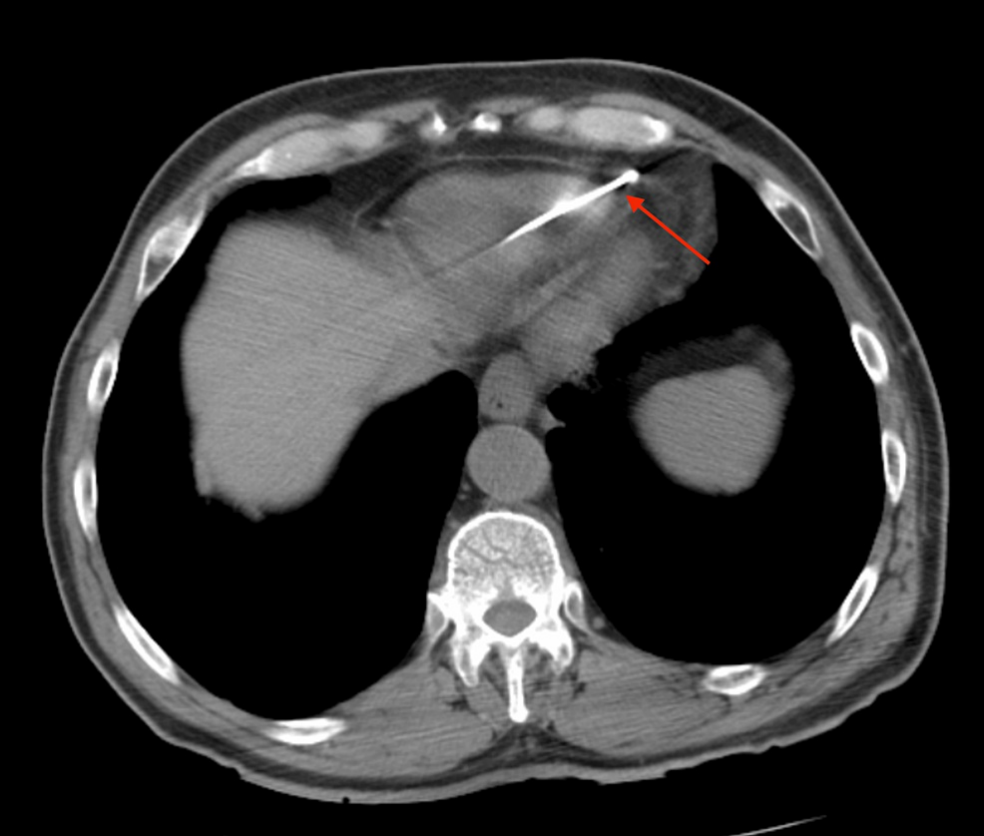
CT chest on presentation CT chest on presentation shows the right ventricular pacemaker lead perforating the right ventricle and the tip of the lead in the pericardium (red arrow).
